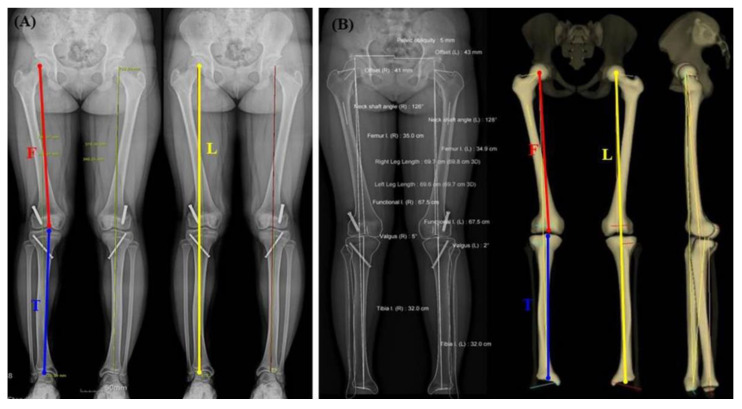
(A) Standing anteroposterior radiograph of the lower extremities on the CR-based teleoroentgenogram, demonstrating measurements of the leg (L), femur (F) and tibia (T) lengths, and hip–knee–ankle angle (HKA). (B) Standing anteroposterior and lateral radiograph of the lower extremities on EOS^®^, demonstrating measurements of the leg (L), femur (F) and tibia (T) lengths, and hip–knee–ankle angle (HKA).
